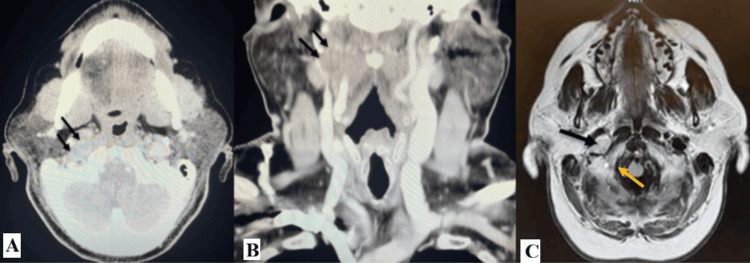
(A) An axial CT image of the head and neck showing an enlarged, well defined, hypoechoic mass. (B) Coronal view of CT imaging showing the same hypoechoic mass. (C) An axial MRI of the brain revealed a 3.2 cm × 1.7 cm mass located near the hypoglossal canal extending into the right neck. The yellow arrow indicates the medial opening of the hypoglossal canal
